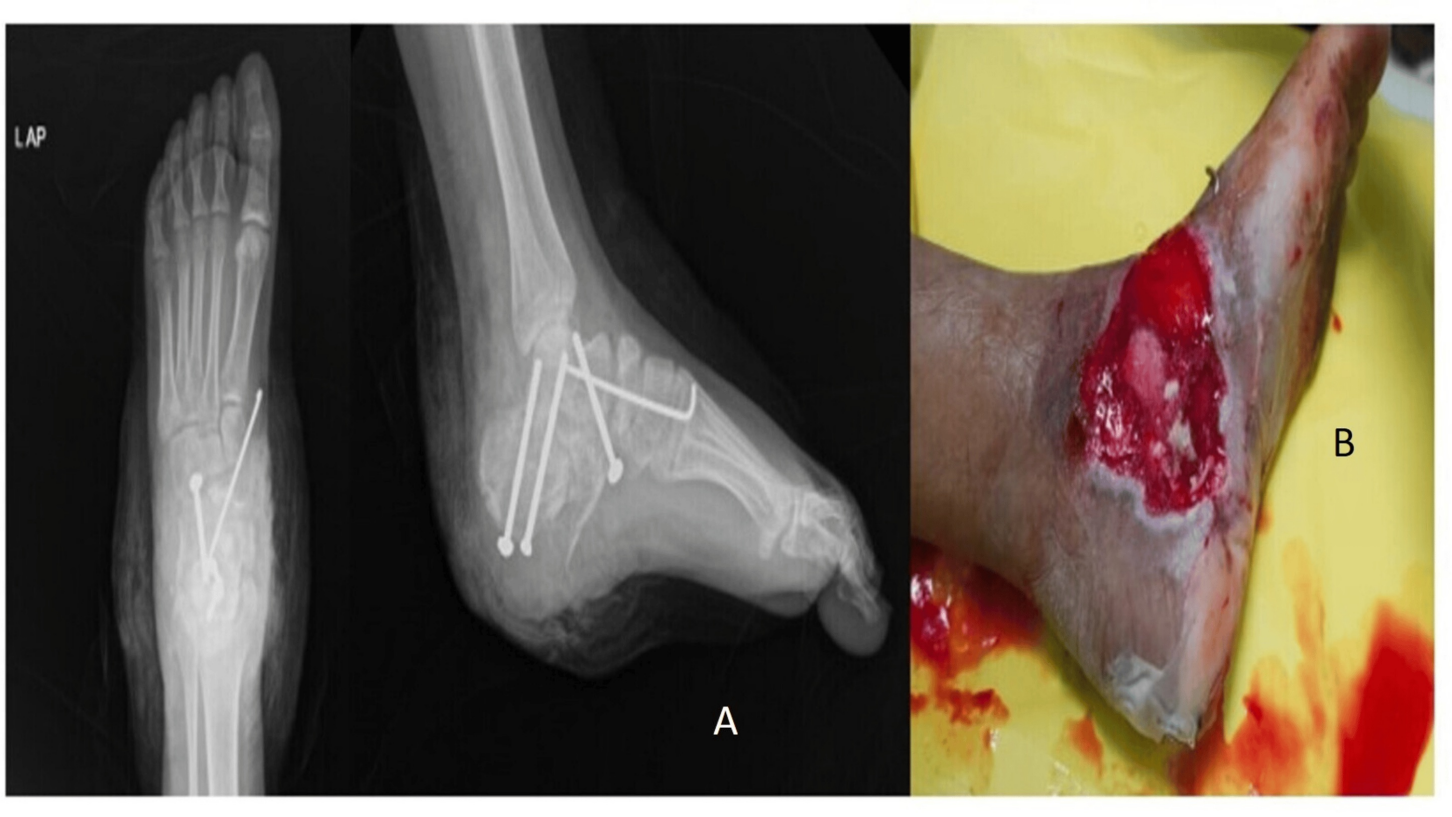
Postoperative radiograph and post-vacuum-assisted closure wound. (A) Postoperative anteroposterior and lateral view of foot radiograph showing fixation with screws and dislocation reduced by K-wire. (B) Wound post-debridement and vacuum-assisted closure application for seven days showing healthy granulation tissue.
